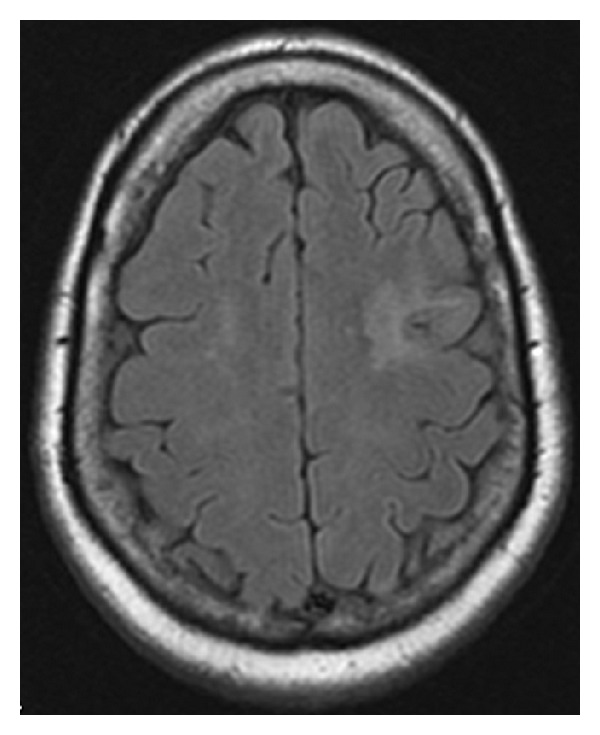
MRI Brain-axial T2 FLAIR showing left frontal lesion.
